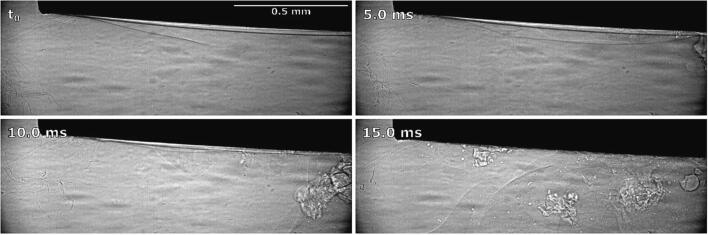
Synchrotron observation of emulsion formation from thin oil layer underneath ultrasonic horn with the gradual disappearance of aforementioned oil layer.
